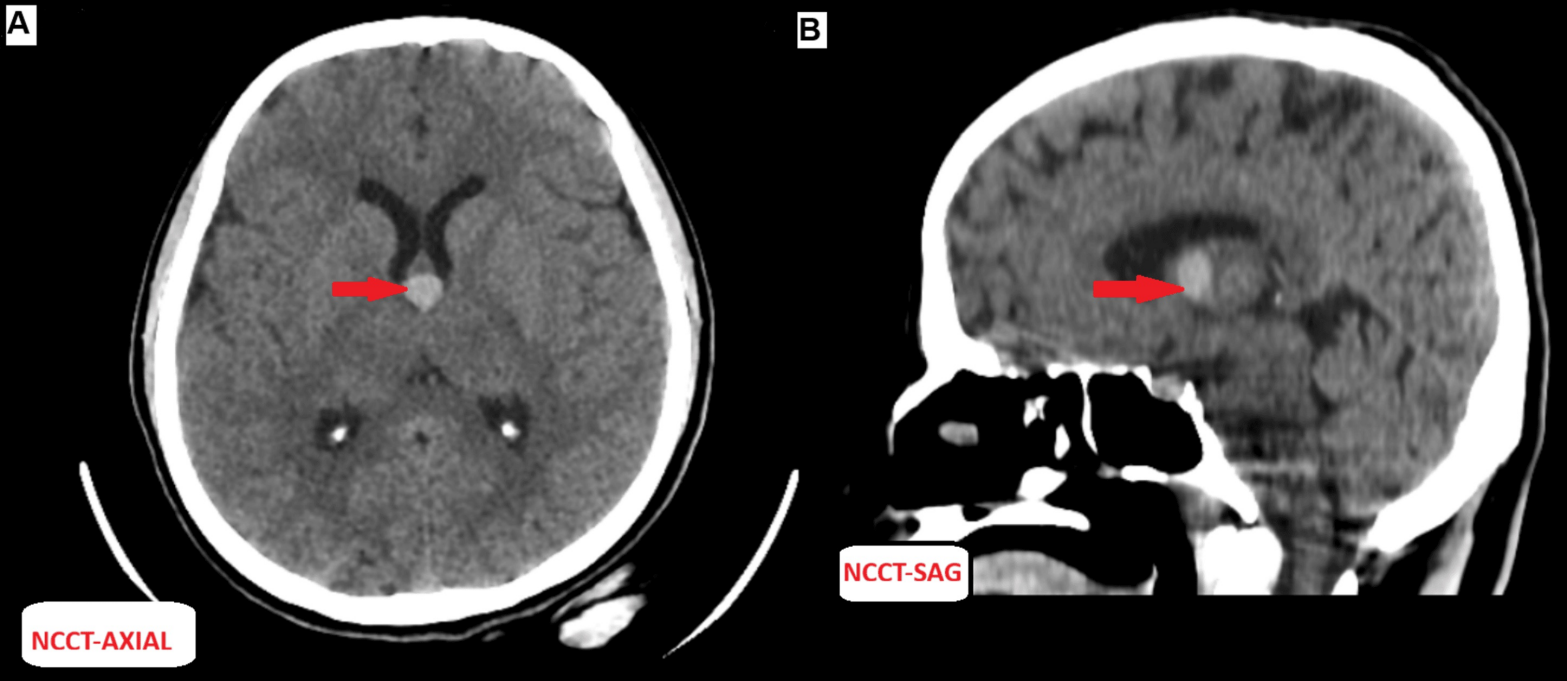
NCCT brain images: (A) axial section and (B) sagittal reformatted image (A) Axial NCCT shows a well-defined hyperdense cystic lesion near the foramen of Monro (red arrow), measuring approximately 9.4 × 9.0 mm (HU: 47). (B) Sagittal section confirms the lesion’s location, with no associated hydrocephalus or CSF flow obstruction, consistent with an incidental finding. Note: All images are original and obtained from the institutional study dataset. NCCT, non-contrast CT
